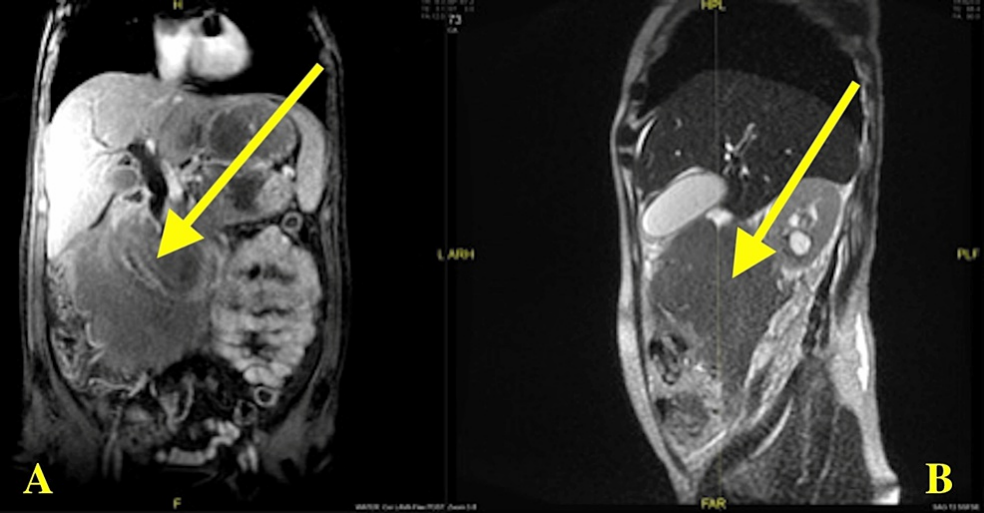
MRI abdomen and pelvis with panels A and B showing two large peritoneal masses displacing the pancreas anteriorly, spleen laterally, and the right kidneys inferiorly. MRI, magnetic resonance imaging
